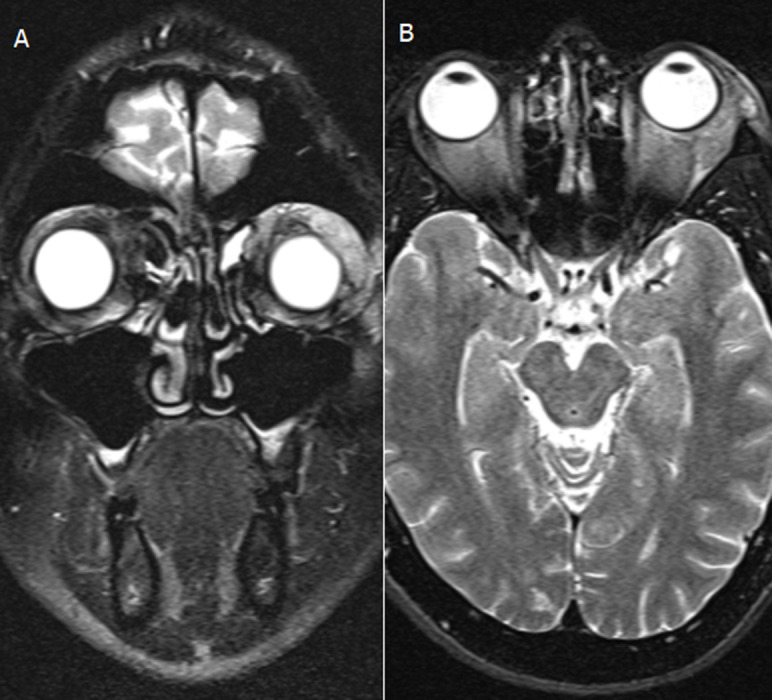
T2W magnetic resonance imaging of the brain and orbit; A) coronal cut showing enhancement and enlargement of the left lacrimal gland; B) axial cut showing enhancement and enlargement of the left lateral rectus and surrounding soft tissue
